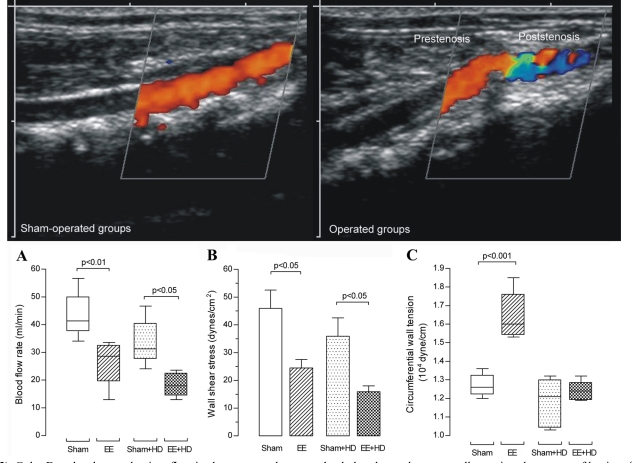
Color Doppler shows a laminar flow in sham-operated groups, the dark red near the aorta wall meaning slower rate of laminar flow.
